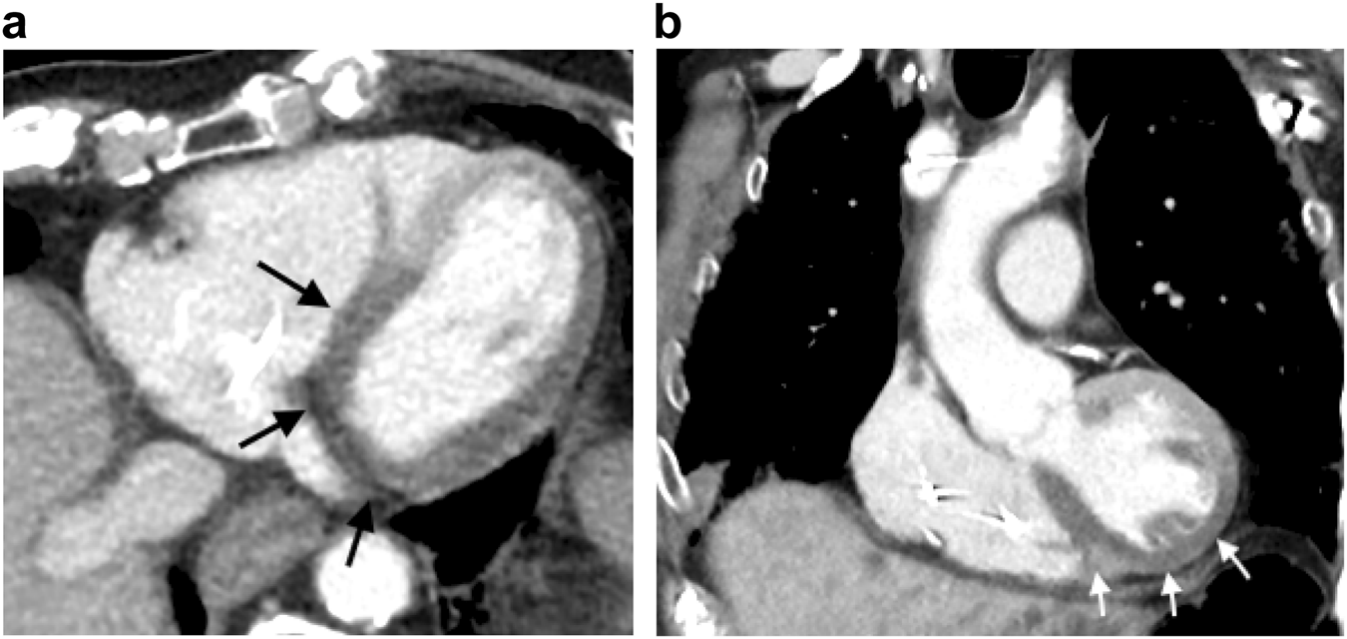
Acute inferior myocardial infarction as a differential diagnosis of acute abdominal pain. Axial and coronal portal phase CT (a, b) in a 90-year-old man shows a segmental perfusion defect of the inferior myocardia (arrows) corresponding to the territory of the circumflex artery, suggestive of an acute infarction
